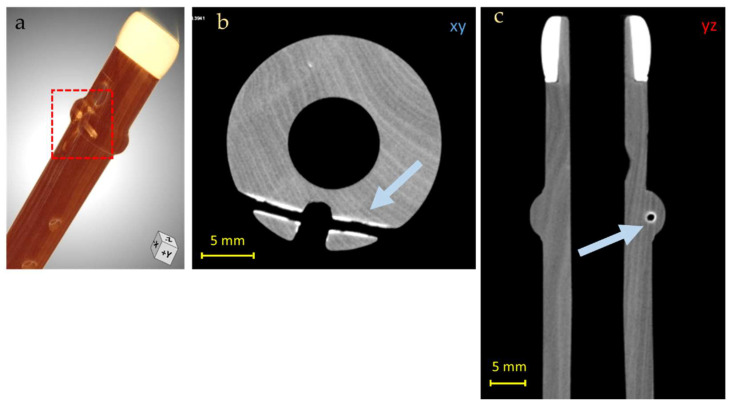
3D render of the Piccolo Flute foot (a) and CT horizontal (b) and lateral (c) slices of the Piccolo Flute foot, in which the supposed metallic residues from the brass key insertion is observed (blue arrows in (b,c) and red square in (a)).
